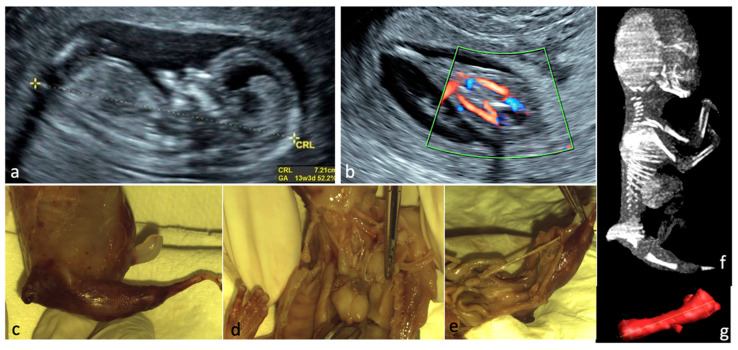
Sirenomelia. (a)—Sagittal view of the fetus at FT anomaly scan, showing normal profile, normal nuchal translucency, and present nasal bone. (b)—Single lower limb, pathognomonic aspect for sirenomelia, two femoral arteries seen. (c–e)—Aspects from conventional autopsy: sagittal macroscopic view of the fetal pelvis, frontal view of the heart and great vessels (right lung removed), bilateral absent kidneys. (f)—CT scan, sagittal view of the fetus (CT scan performed after obtaining the autopsy data). (g)—Single femur measurements, Cone Beam CT technique used.
